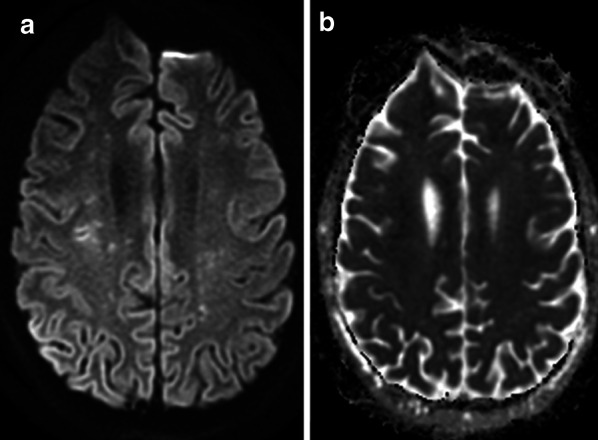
A case representing the starfield pattern of restricted diffusion. MRI scan of an 18-year-old man with a closed displaced fracture of the left femoral shaft after a high velocity motor vehicle accident. Diffusion-weighted MR Image (10000/89; b value, 1000 s/mm^2^) showing foci of hyperintensities within both centrum ovale (a), and the corresponding ADC map confirming restricted diffusion (b). Published under the permission of G. Bierry and S. Kremer, Department of Radiology, University Hospital of Strasbourg, Strasbourg, France
